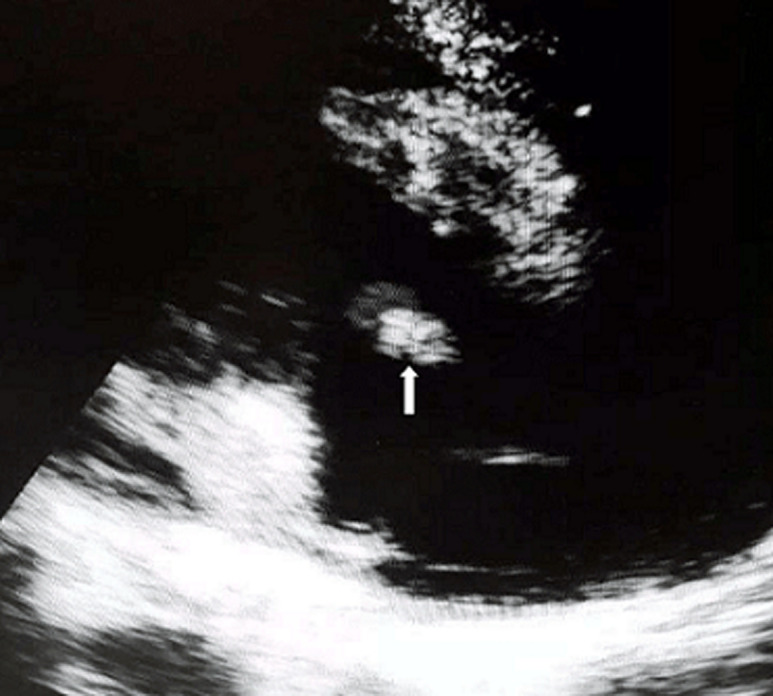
transthoracic echocardiography showing vegetation on anterior leaflet of tricuspid valve (white arrow) on apical four chamber view
